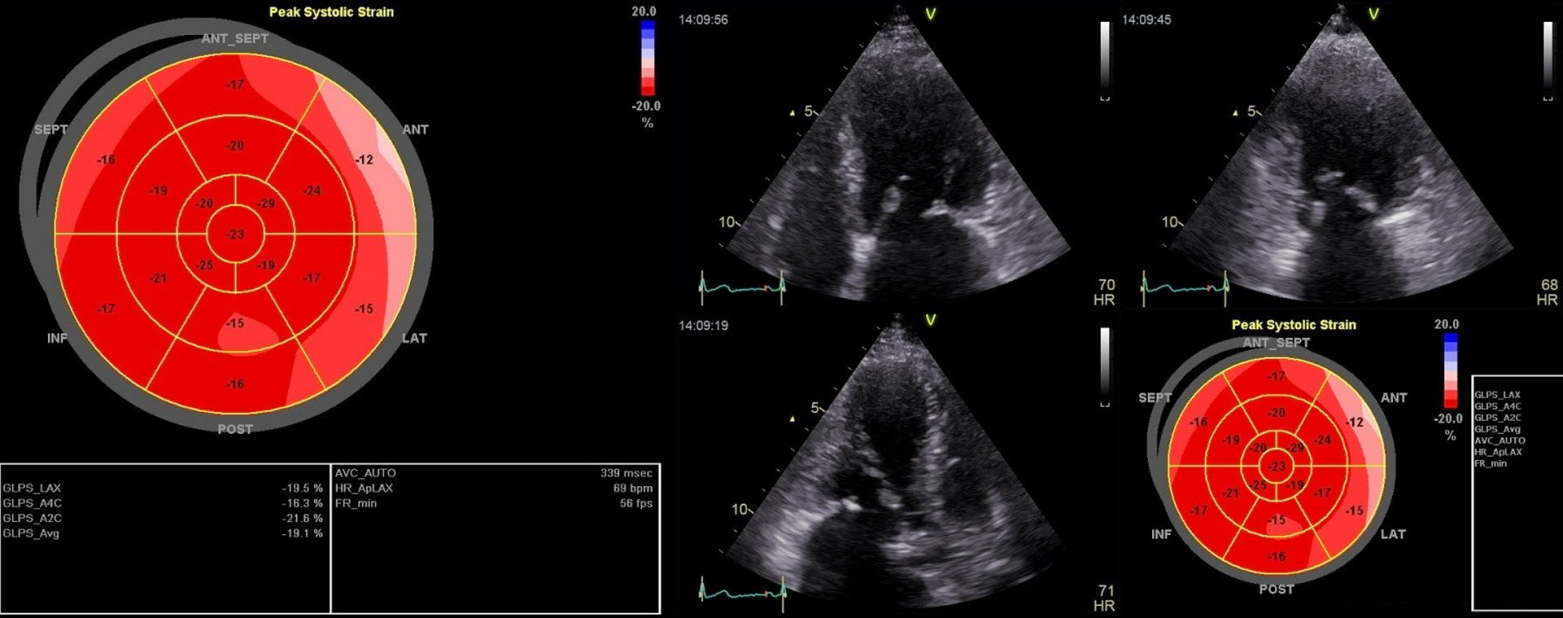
Bull’s eye plot in a patient with severe rheumatic MS showing reduced segmental longitudinal strain values in basal and some mid myocardial segments despite good global longitudinal strain. As presented in the image, three 2-D echocardiographic views, including 4, 3, and 2 chamber apical views with a focus on LV, were used to assess global and longitudinal strain values. To perform, in each view, three points were plotted manually at the base of each opposing wall as well as the apex using a point-and-click technique, and then the endocardial border was automatically traced by the software. The software divides the region of interest in each view into six segments, and the quality of tracing of each segment is automatically scored as either acceptable or unacceptable with the possibility of further correction manually. Finally, the software calculates global and segmental strains, displaying the values. (The figure is only for demonstration of the technique and was not taken from an included patient in the study)
